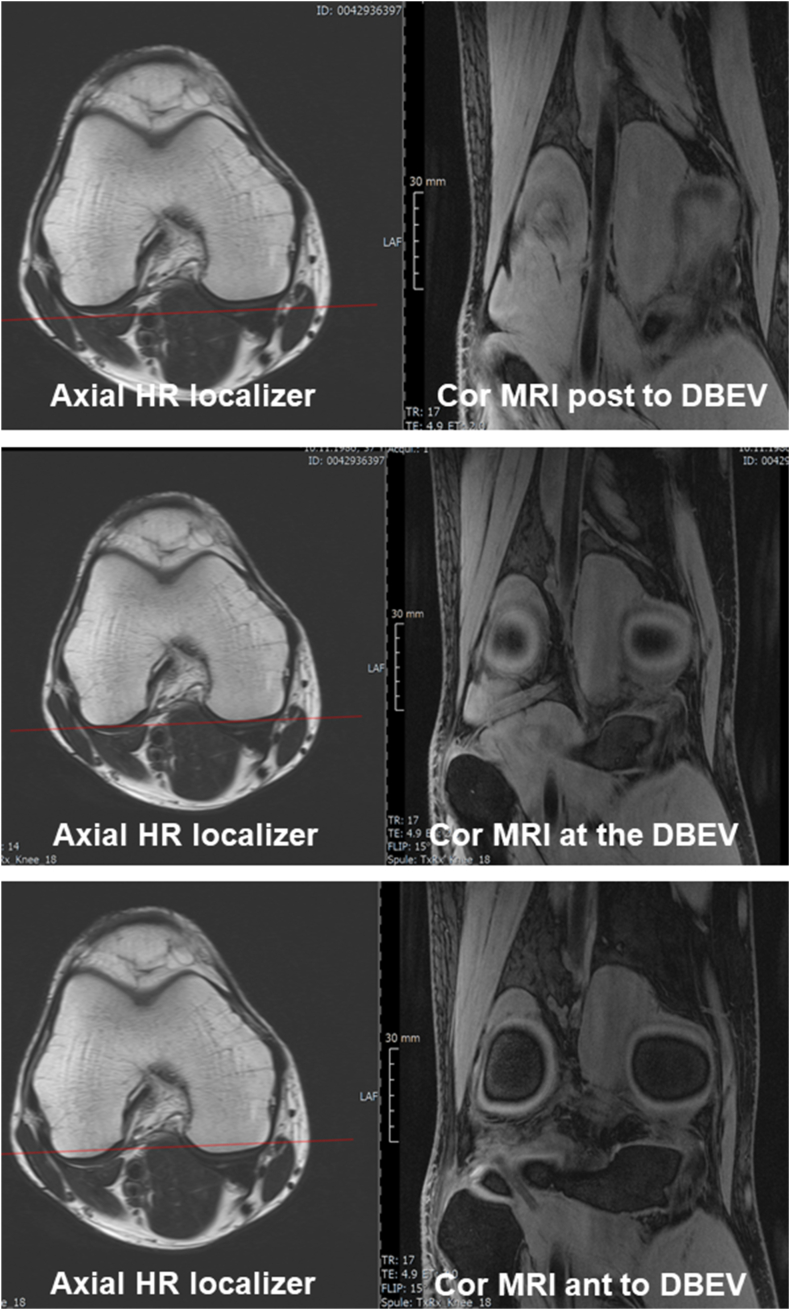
Axial HR localizer images shown on the left with a red line displaying the position of the respective coronal MRI. The middle image on the right shows a coronal MRI positioned double oblique to obtain a double bull eyes view (DBEV). The superior image on the right shows a coronal MRI posterior, and the inferior image a coronal MRI anterior to the DBEV. The MRIs were obtained on a 3T Siemens VIDA scanner.
